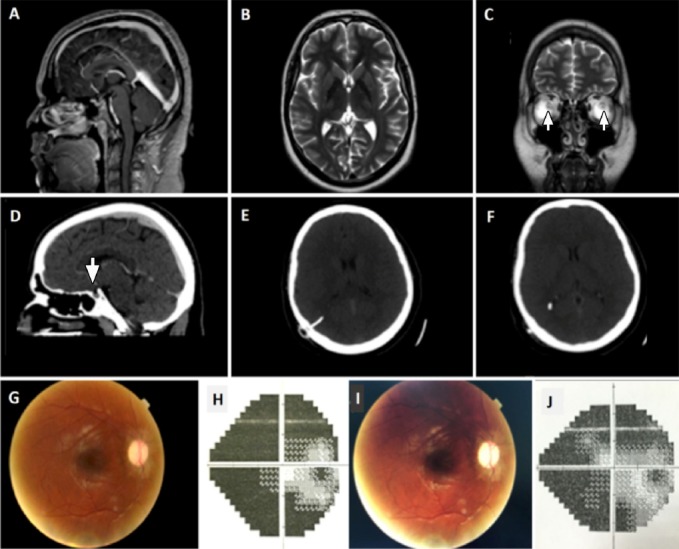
Imaging, fundoscopic pictures and visual field of the patient A) Sagittal T2-weighted MRI. B) Unremarkable axial T2-weighted MRI. C) Coronal T2-weighted MRI image demonstrates bilateral widening of the optic nerve sheath. D) CT venogram of the patient showing a partially empty sella. E, F) CT of the brain, 1 day postoperatively, demonstrating VP shunt insertion. G) Fundoscopic picture showing improved optic disc edema in the patient’s right eye (3 months postoperatively). H) Humphrey visual field test showing residual constriction of the patient’s peripheral vision in the right eye (3 months postoperatively). I) Fundoscopic picture of the patient’s right eye (7 months postoperatively). J) Humphrey visual field test showing some improvement of the patient’s peripheral vision in the right eye (7 months postoperatively).
